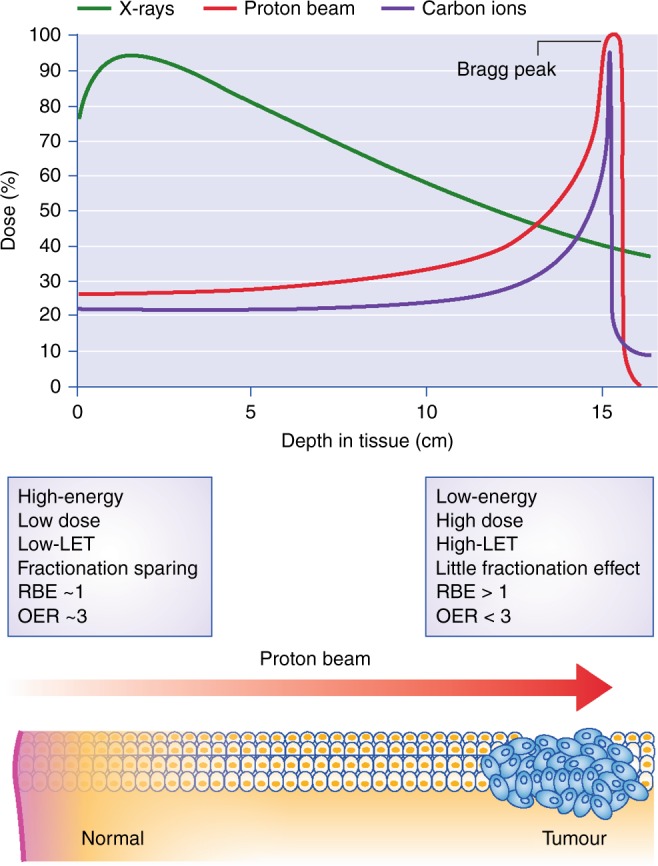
Particle therapy exploits a favourable depth–dose curve. Charged particles deposit most of their initial energy when they slow down, close to the end of the range (Bragg peak) within the tumour target, while X-ray energy decreases exponentially with dose. Particles at high energy (entrance, normal tissue) have linear energy transfer (LET) similar to X-rays, while the LET becomes high when the ions slow down around the Bragg peak. This provides radiobiological advantages such as an increased relative biological effectiveness (RBE) and reduced oxygen enhancement ratio (OER) in the tumour
